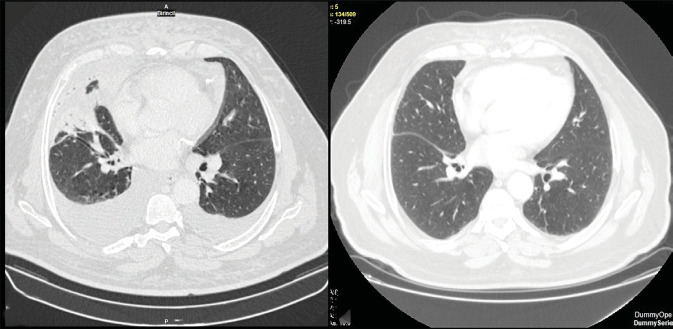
Figure 2. (A) Thorax computed tomography revealed
